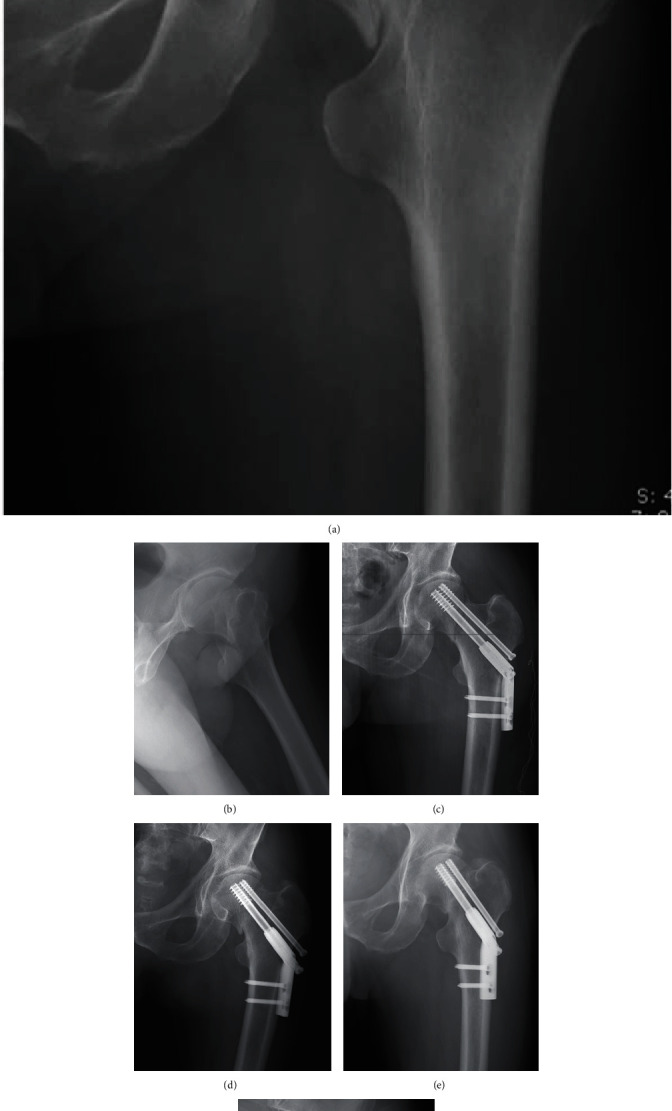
Follow-up of a 63-year-old male patient with FNF. (a) Preoperative AP radiograph. (b) Preoperative lateral radiograph. (c) Radiograph immediately after surgery showing an anatomical reduction of fracture. (d) Radiograph at 4 weeks postoperatively showing the anatomical reduction changed to negative buttress position. (e) AP radiograph at 6 months postoperatively showing femoral neck shortening. (f) Radiograph at 24 months after surgery showing the femoral neck fracture healed in a deformed position.
